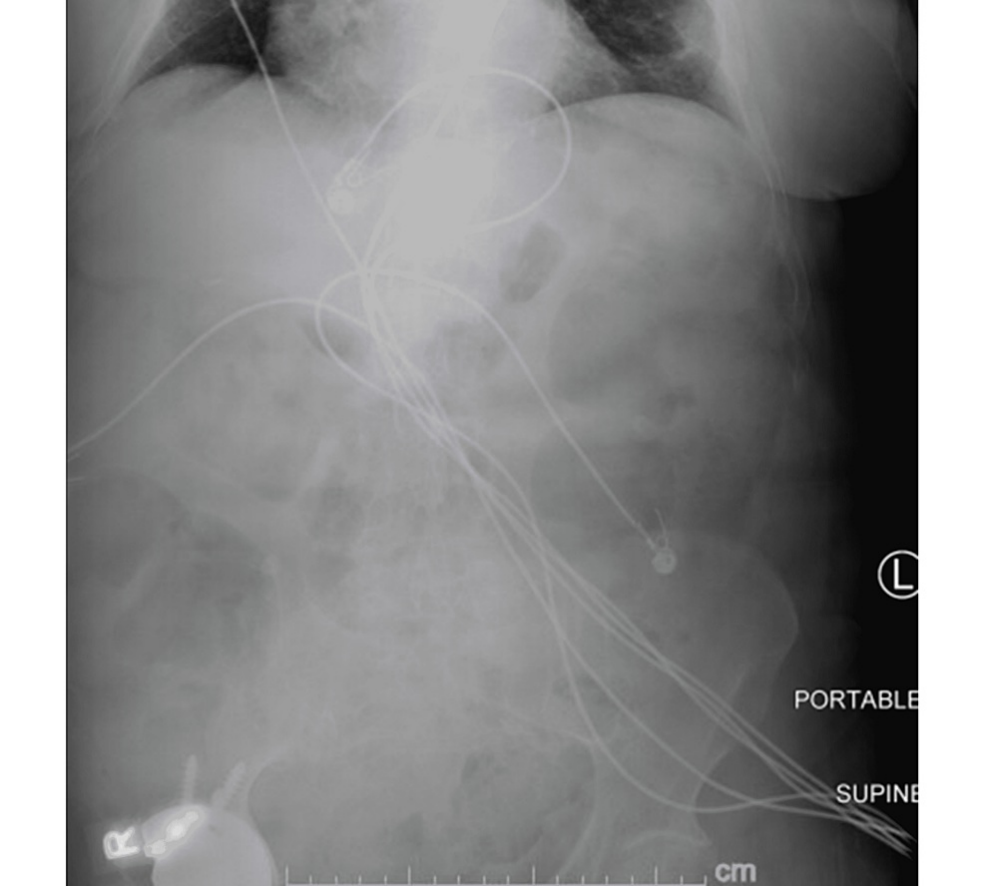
Abdominal X-ray showing no evidence of free intraperitoneal air, dilated colon, or obstruction.
